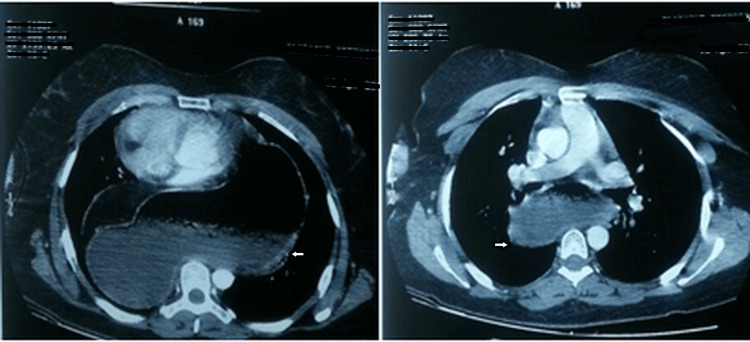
Diaphragmatic hernia and intrathoracic gastric fluid distension on thoraco-abdominal CT scan
